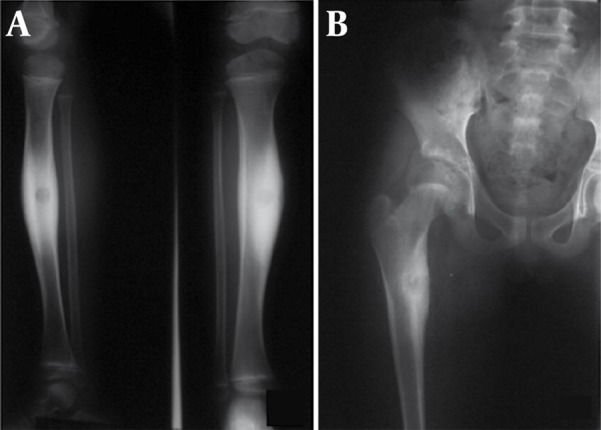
A, A 14-Year-Old Man With Leg Pain; and B, An 18-Year-Old Female With Pain in Her Thigh. In the plain film the nidus is calcified and surrounded by increased bone density.
